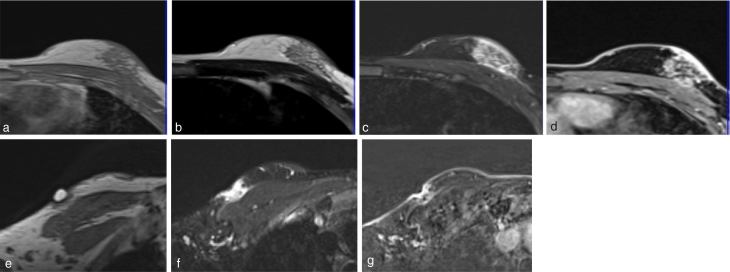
MRI of the left breast depicts isointense focal area of marked fat stranding with overlying skin thickening in the outer half of the left breast that is isointense on *T*
